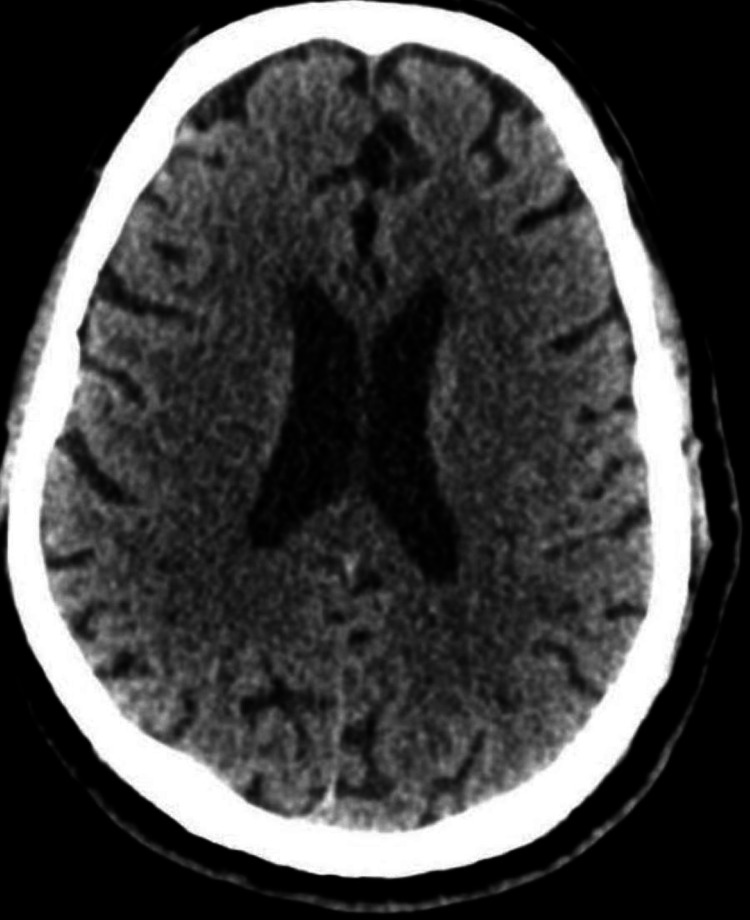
Plain CT scan of the brain with normal parenchyma and normal ventricles
